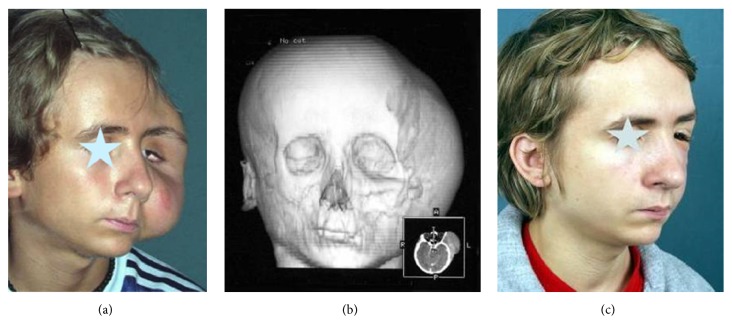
Patient with neurofibroma of the face (a), CT (b), and the case after tumor resection (c).
